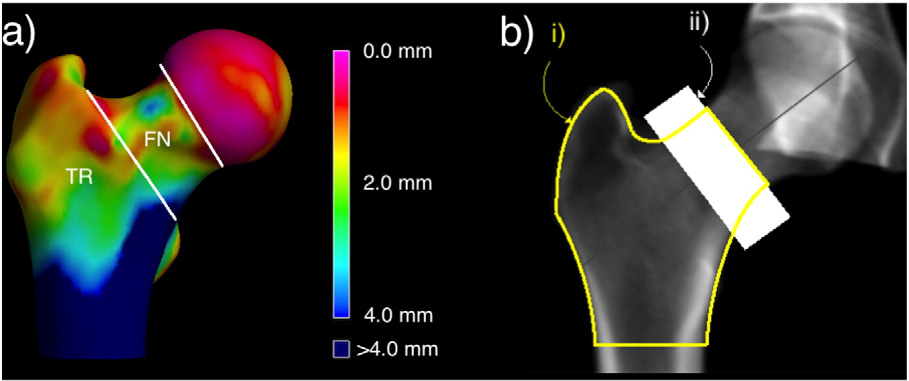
a) Boundaries for classifying the two main types of hip fracture; femoral neck (FN) and trochanteric (TR), shown on a 3D Bone Map of cortical thickness from a clinical CT scan b) Standard clinical two-dimensional areal bone mineral density (aBMD) measurements of i) the total hip region and ii) the femoral neck region. Approximate boundaries for aBMD measurement used in this study (QCTpro CTXA version 5.1.3, Mindways Software Inc., Austin, Texas).
